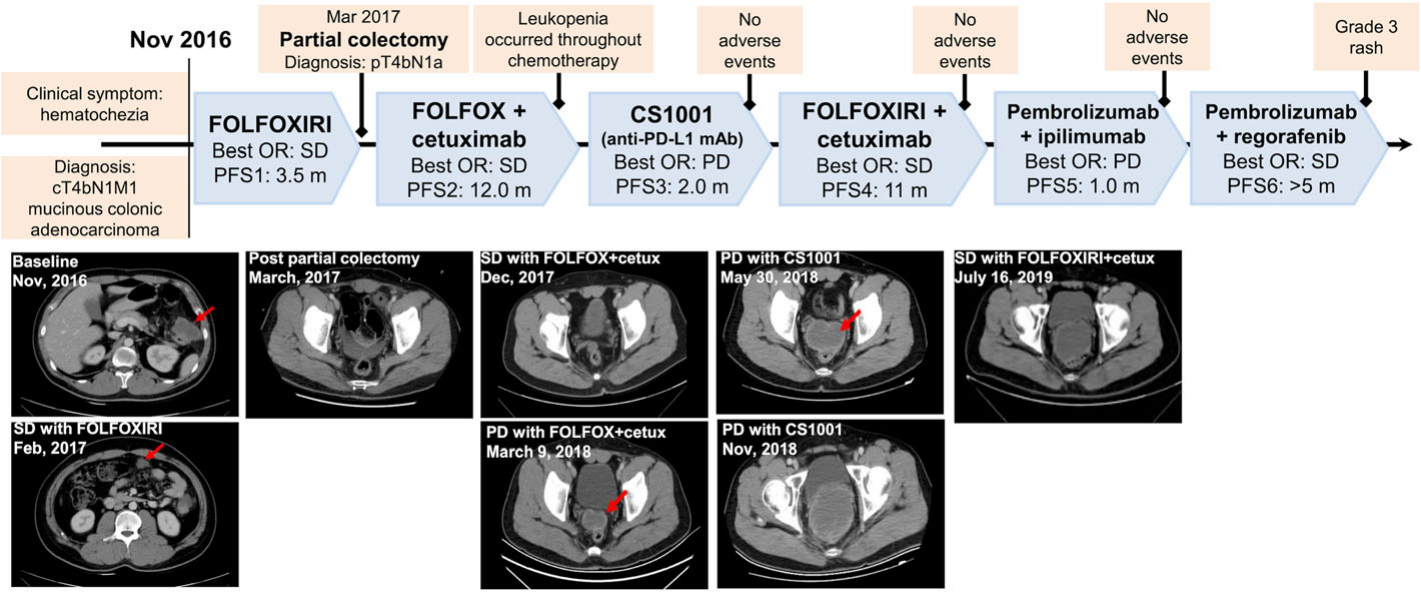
Summary of the treatment received by the patient, including the best objective response (OR) and progression-free survival (PFS) in each line of treatment. Abdominal computed tomography (CT) scans are also provided. Red arrows indicate the primary lesions
